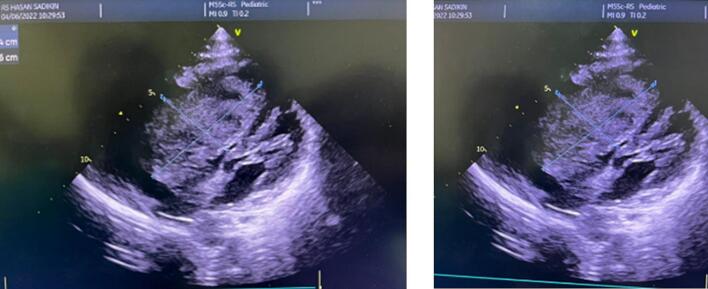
Transthoracic echocardiography reveals a mass filling the right atrium and right ventricle at PA.
